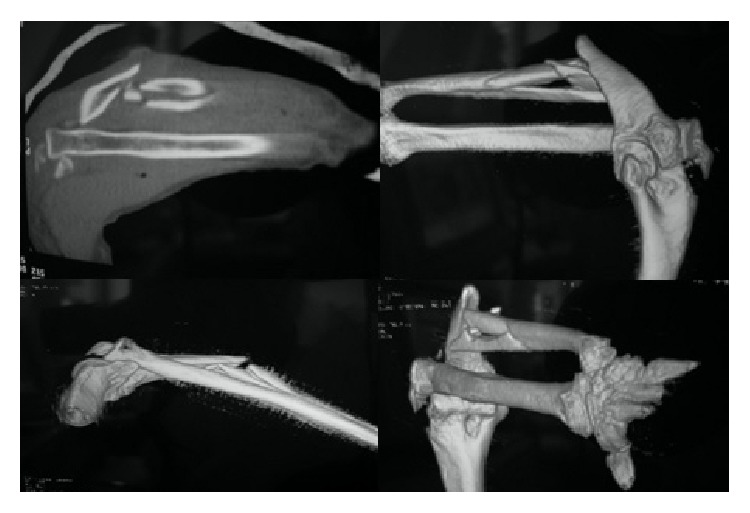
CT-scan with 3D reconstruction.
